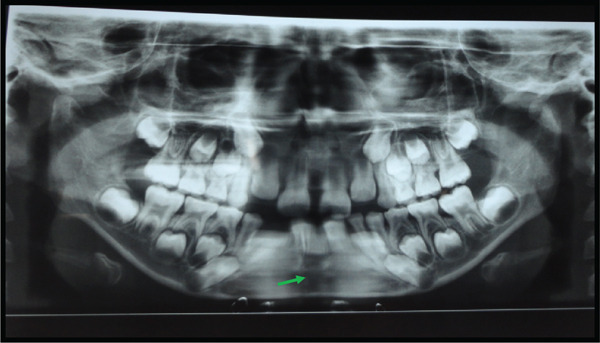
Orthopantomograph of the jaw.
